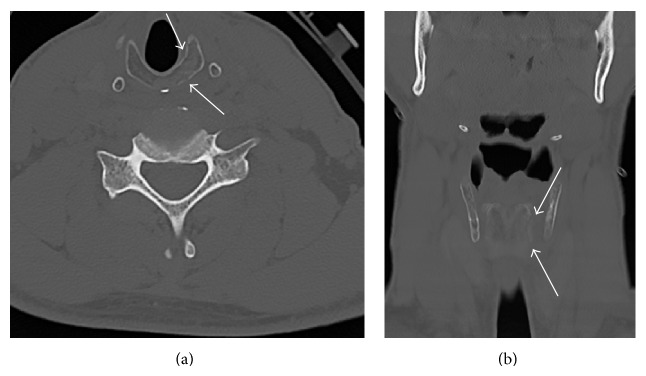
(a) Case B: axial noncontrast CT of the cervical spine shows acute comminuted fracture of the left aspect of the cricoid cartilage. (b) Case B: coronal noncontrast CT of the cervical spine shows acute fracture of the left aspect of the cricoid cartilage.
